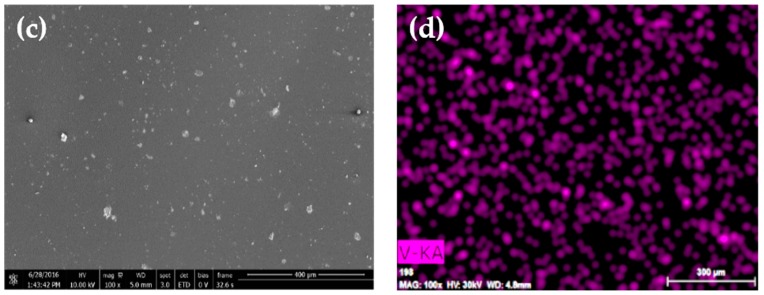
SEM images and X-ray dot map (V-Kα) of the EVA/VO_2_ prepared via melt blending (a,b); and those of the solution casted EVA/VO_2_ film (c,d).
